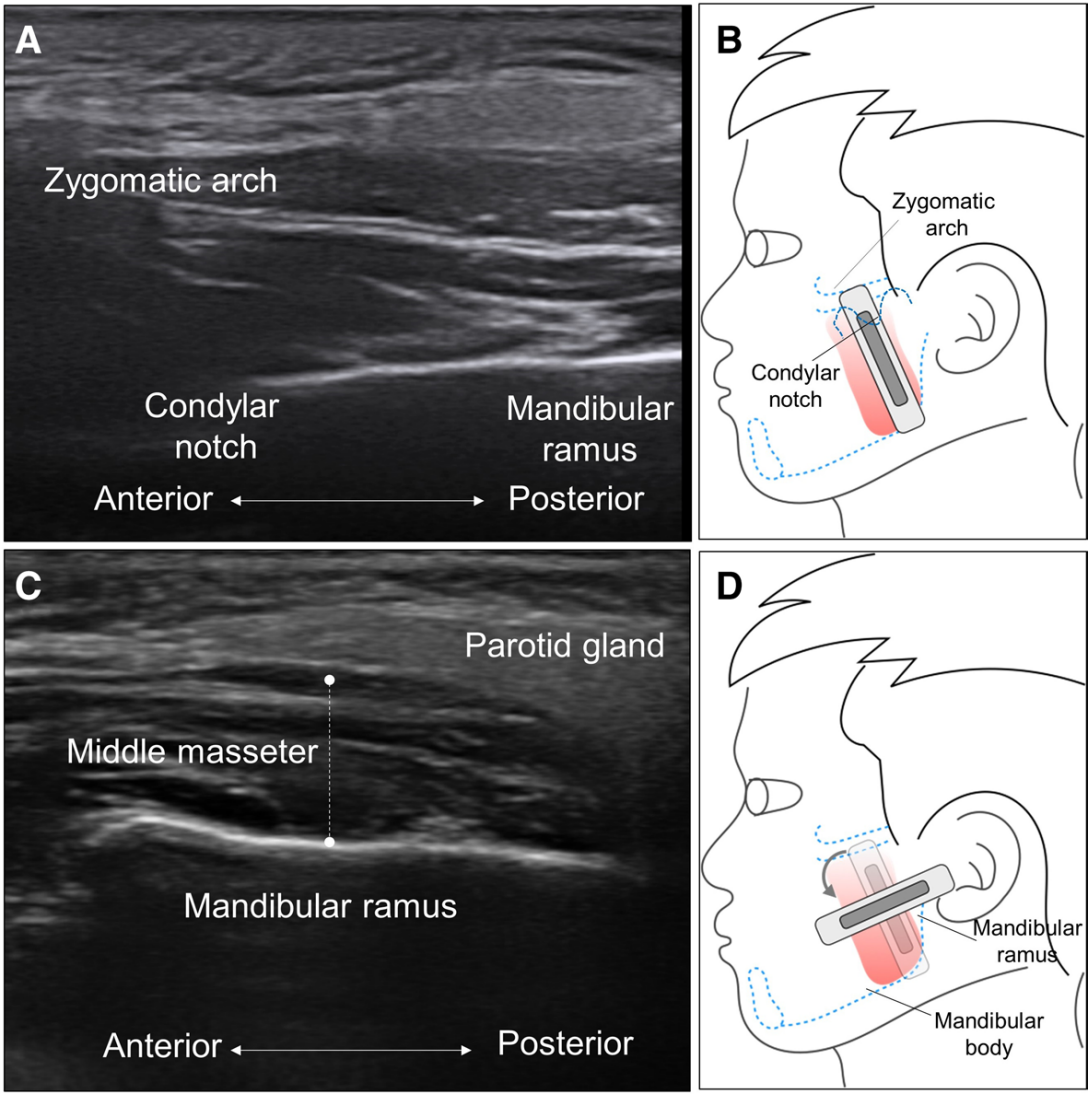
Ultrasound imaging (A) when the transducer is placed in the coronal plane bridging the condylar notch (B). Ultrasound imaging (C) of the middle masseter muscle when the transducer is rotated to the horizontal plane (D). Note that the length of the dashed lines indicates the thickness of the muscle.
